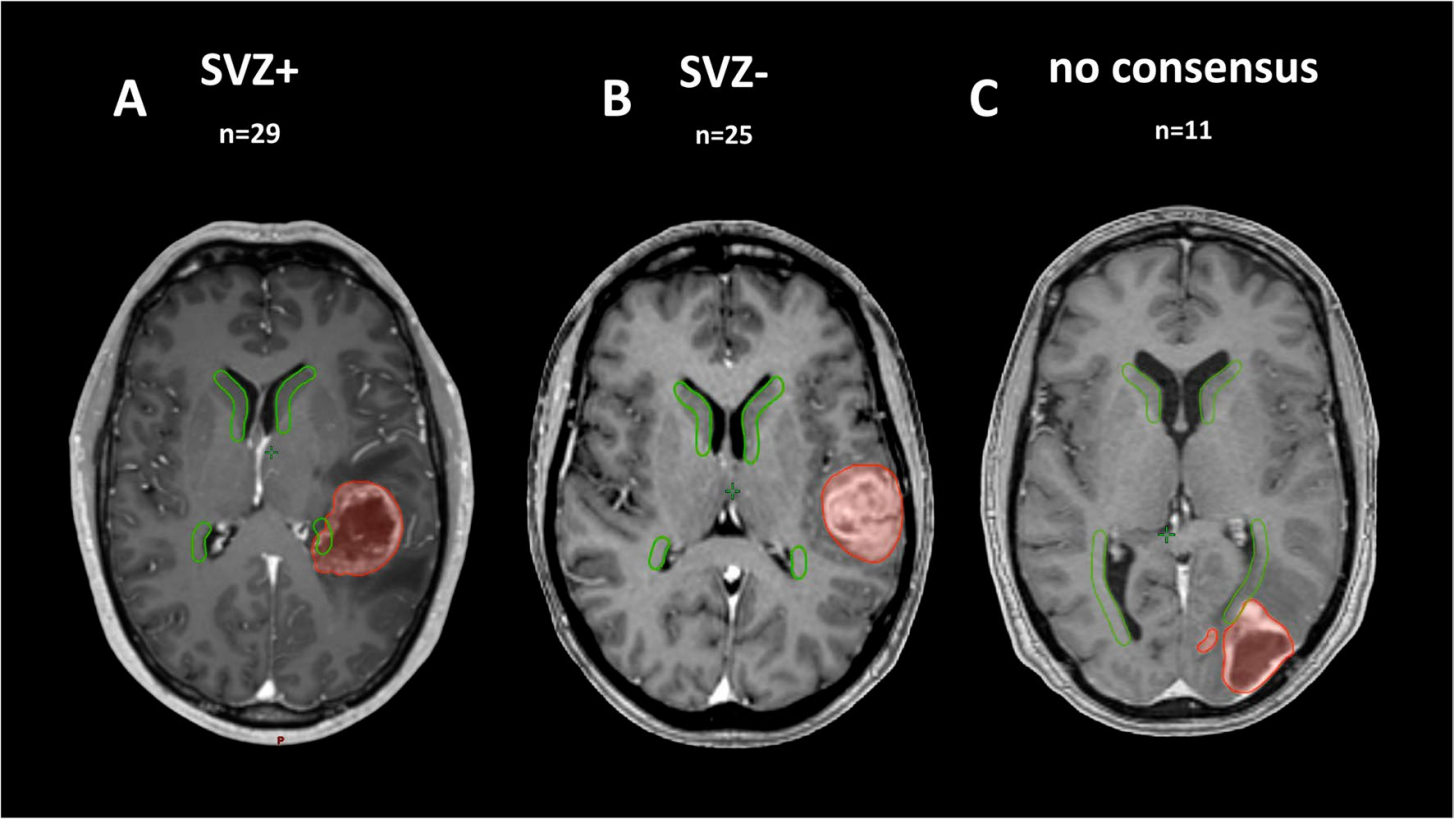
Representative axial T1ce MRI for SVZ contact assessment and three examples. A SVZ + , B SVZ-, and (C) missing consensus amongst readers. SVZ = green, GBM = red
